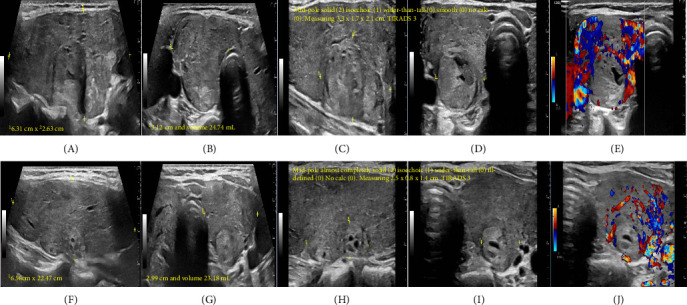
Initial thyroid ultrasound when patient has thyroid storm. (A) Right (R) thyroid lobe in longitudinal axis with heterogenous echogenicity and measuring^1^ 6.31 cm × ^2^2.63 cm. (B) R thyroid lobe in transverse axis with heterogenous echogenicity measuring^1^ width 3.12 cm and volume 24.74 mL. (C) R thyroid mid-pole nodule in longitudinal axis measuring^1^ 3.3 cm × 21.7 cm. (D) R thyroid mid-pole nodule in transverse axis and measuring^1^ 2.1 cm. Mid-pole, solid (2), isoechoic (1), wider-than-tall (0), smooth (0), and no calc (0). Measuring 3.3 cm× 1.7 cm × 2.1 cm. Points: 3. TIRADS 3. (E) R thyroid lobe doppler in transverse axis with hypervascularity. (F) Left (L) thyroid lobe in longitudinal axis with heterogenous echogenicity measuring^1^ 6.56 cm ×^ 2^ 2.47 cm. (G) L thyroid lobe in transverse axis with heterogenous echogenicity and measuring width^1^ 2.99 cm and volume 23.18 mL. (H) L thyroid mid-pole nodule in longitudinal axis and measuring 1 2.5 cm× 2 0.8 cm. (I) L thyroid nodule in transverse axis measuring^1^ 1.4 cm. Mid-pole, Almost completely solid (2,) isoechoic (1), wider-than-tall (0), ill-defined (0), and no calc (0). Measuring 2.5 cm × 0.8 cm × 1.4 cm. Points: 3. TIRADS 3. (J) L thyroid lobe doppler in transverse axis with hypervascularity but less than the R side.
